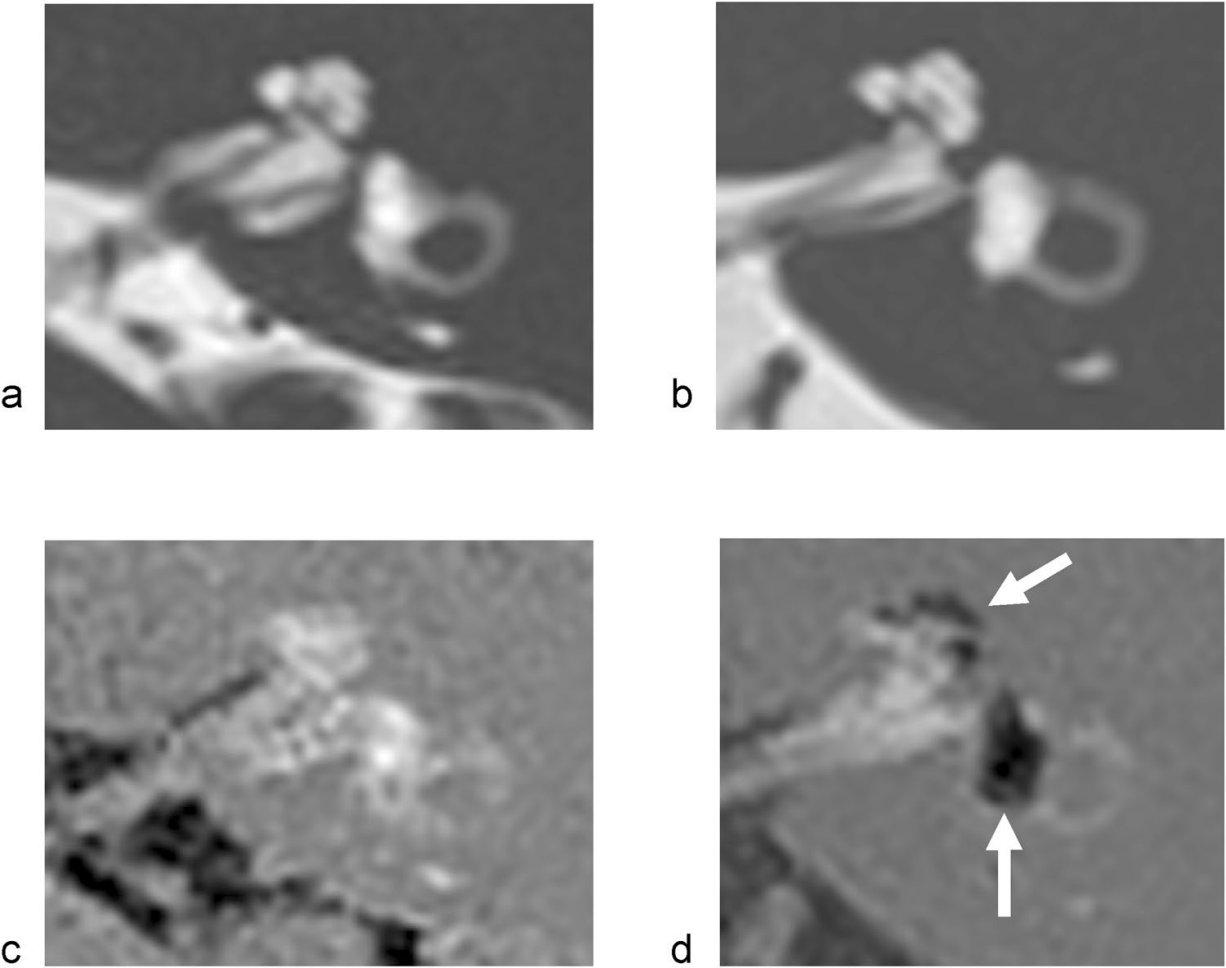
MRI of endolymphatic hydrops in Meniere's disease. a, b MR cisternography, c, d Hybrid of reversed image of positive endolymph signal and native image of positive perilymph signal (HYDROPS) image. Healthy (a, c) and a Meniere’s disease ear (b, d) are presented. MR cisternography (a, b) shows a high signal both for the inner ear labyrinthine endolymph and perilymph. On HYDROPS image obtained 4 h after intravenous administration of single-dose gadolinium-based contrast agent (GBCA) (c, d), endolymph is delineated as low signal since GBCA does not transit into endolymph. In Meniere’s disease ear, dilatation of endolymphatic space in cochlea and vestibule is clearly visualized (d: arrows)
